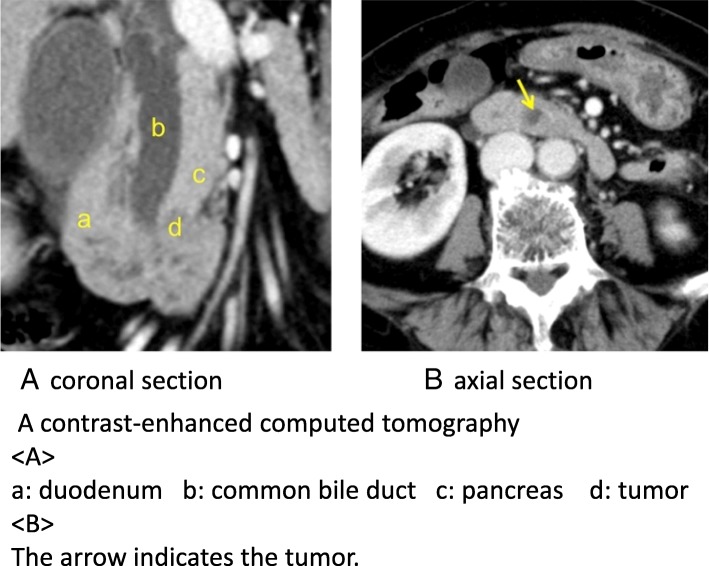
Preoperative computed tomography. Coronal section (A) and axial section (B) of a contrast-enhanced computed tomography scan revealed a hypovascular tumor (arrows) at Vater’s papilla had invaded into the pancreas directly. a, duodenum; b, common bile duct; c, pancreas; d, tumor
